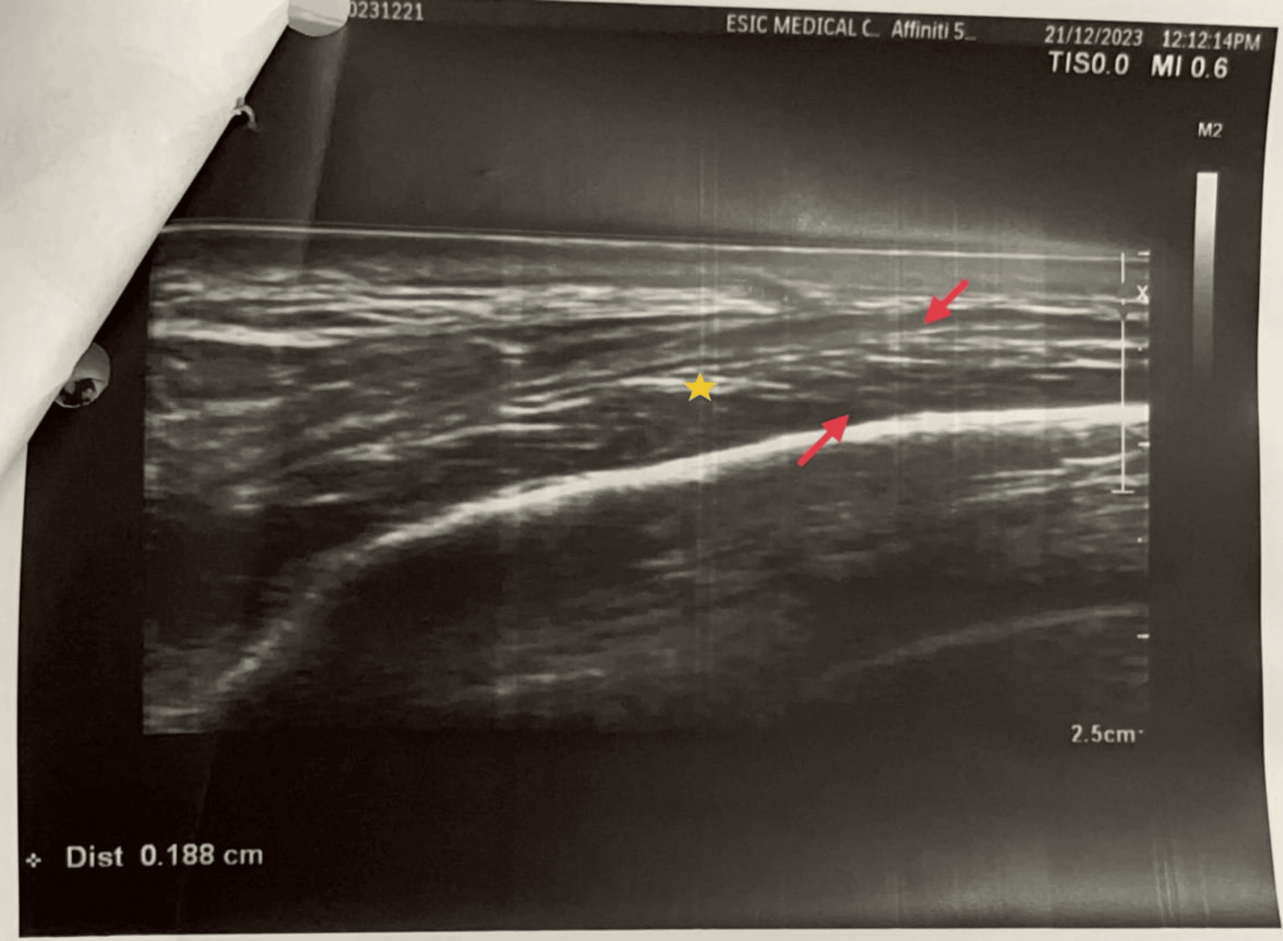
Temporal artery ultrasound The image shows the lumen (yellow star) and the vessel wall (red arrow)
